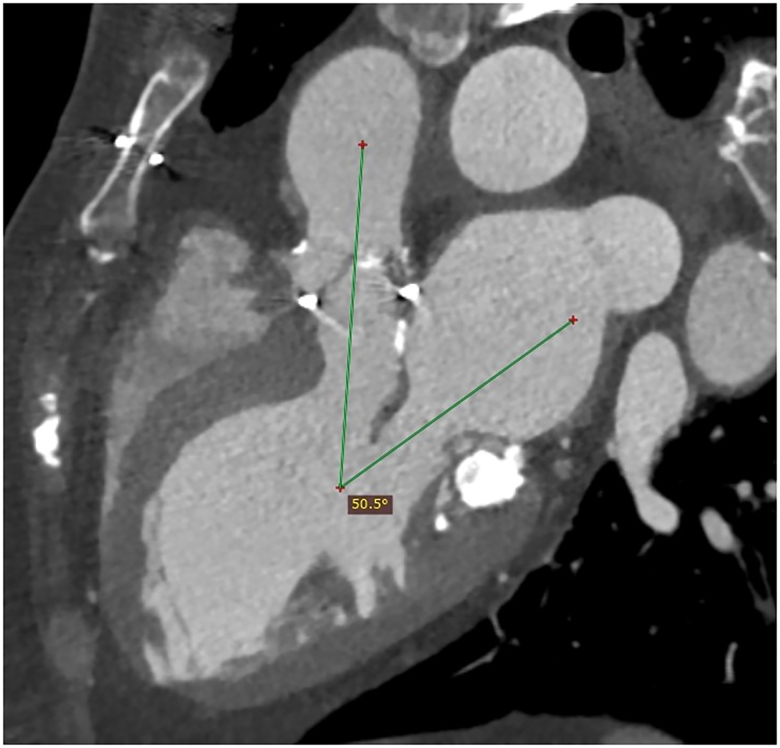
Initial cardiac computed tomography—aorto-mitral angle. Cardiac computed tomography demonstrating acute aorto-mitral angle, posing increased risk for left ventricular outflow tract obstruction. 115 × 120 mm.
